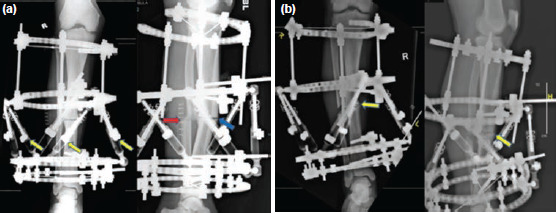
Post-operative radiographs post frame insertion. (a) Immediate post-operation AP and lateral radiographs. Yellow arrows on the AP radiograph indicate the adjustable telescopic struts. The red arrow on the lateral radiograph indicates the oblique osteotomy that was made to the fibula. The blue arrow on the lateral radiograph shows the compression at the fracture site. (b) AP and lateral radiographs at three months post-operation with the hexapod frame still on. The yellow arrows show the bridging callus formation at the fracture sites.
